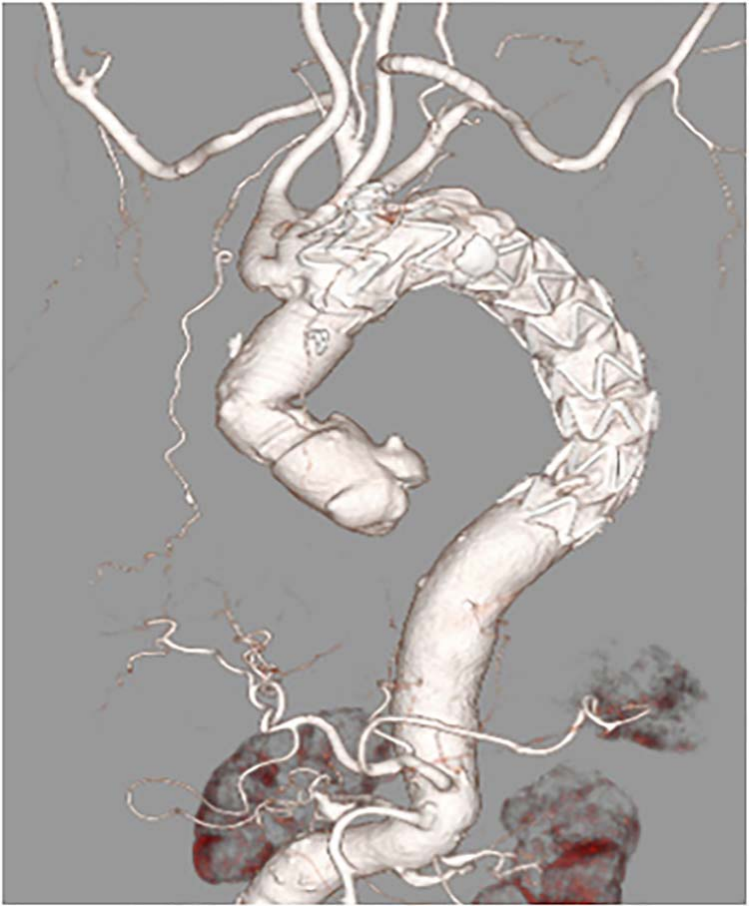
Postoperative enhanced-computed tomography findings. There were no problematic findings, although a slight type 4 endoleak was present.
